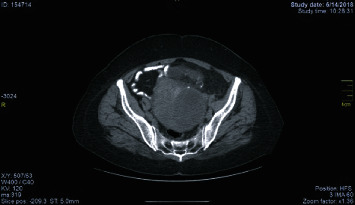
CT scan of the recurrent struma ovarii.
